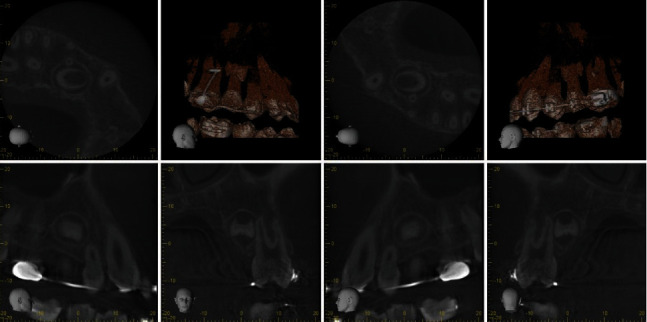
CBCT shows new supernumerary tooth developing in the upper left quadrant.
